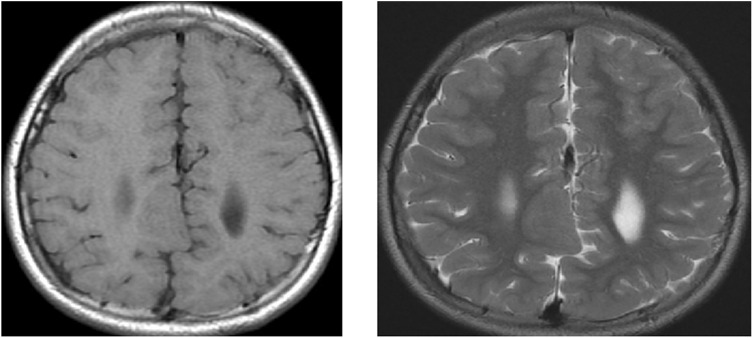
MRI of the brain of a 9-year-old child showing axial T1 weighted image (WI) and axial T2 WI for a large well-defined nodule at the RT subcortical parafalcine region abutting the falx another similar lesion seen at the left frontal region, with both lesions having isointense signals to the gray matter suggestive of gray matter heterotopia.
